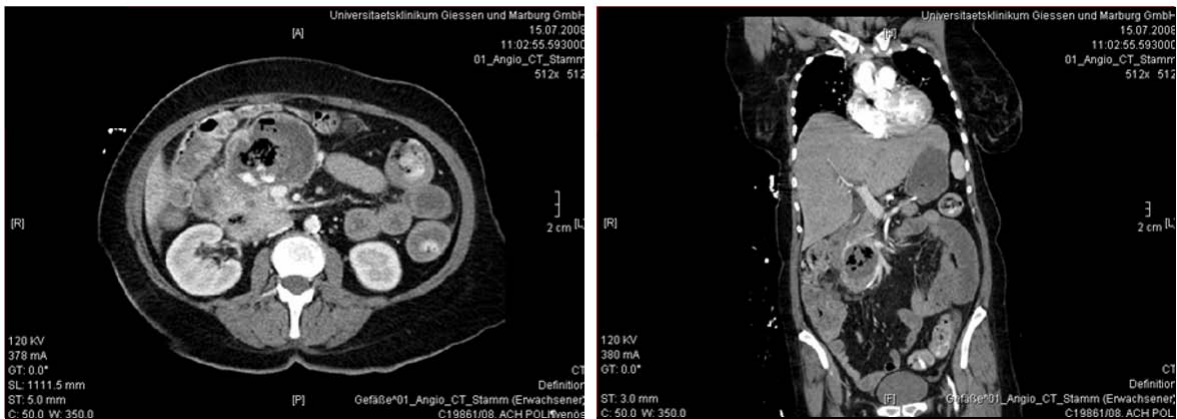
Transversal (left) and coronal (right) CT scans of the abdomen reveal a cystic and necrotic tumor cavity 2 weeks after initiation of imatinib therapy.
